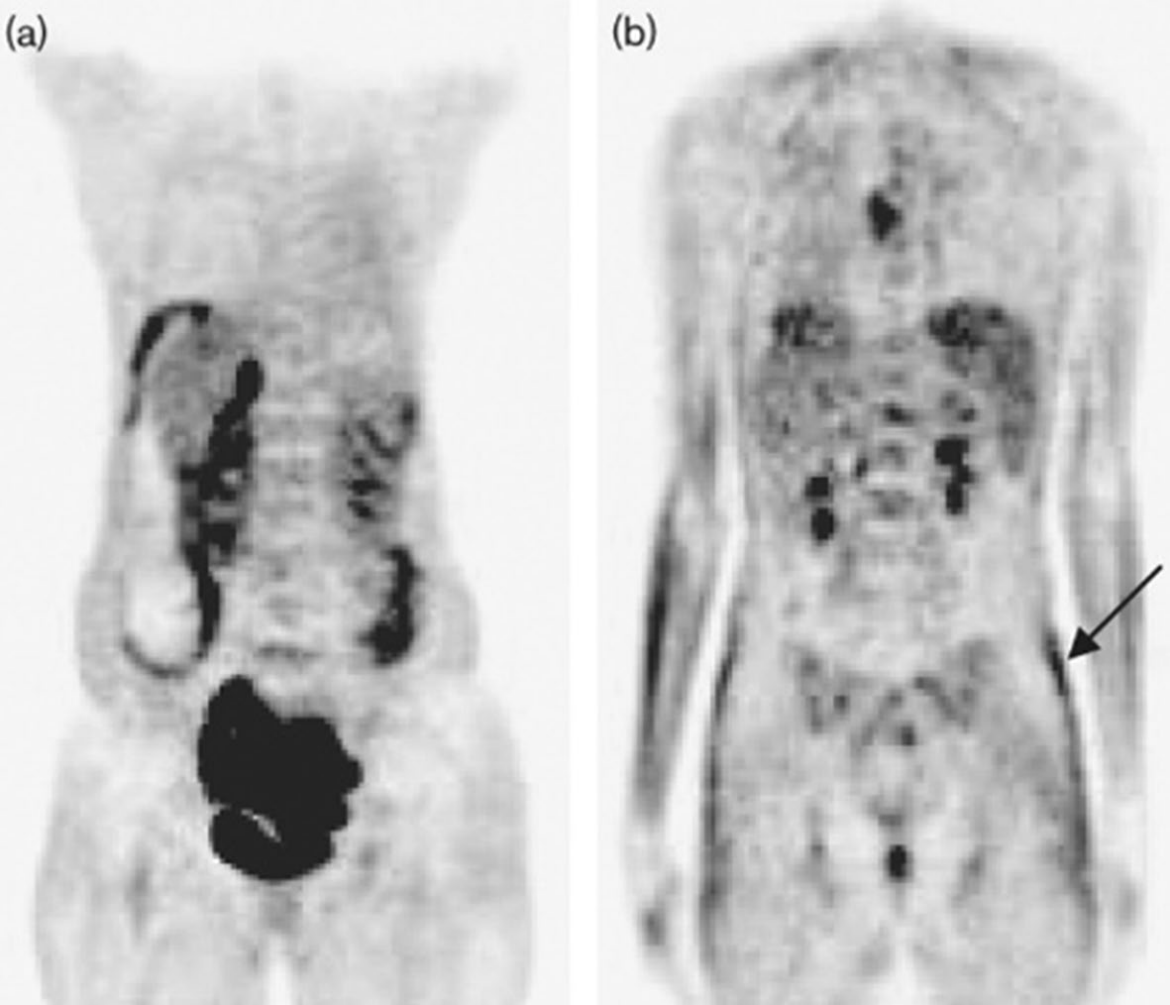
Coronal FDG-PET/CT images of two patients diagnosed with HIV infection and receiving highly active antiretroviral therapy (HAART). While low subcutaneous uptake of FDG is typically observed (A), certain patients with lipodystrophy are found to have increased white fat FDG uptake (B, arrow). Image reprinted without changes from Sathekge et al. (2010)
